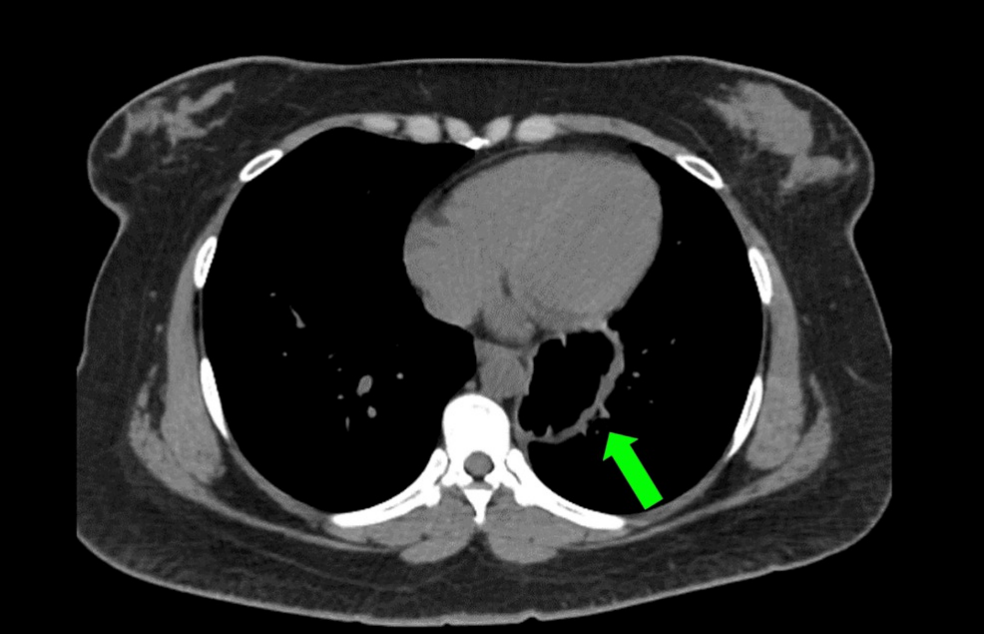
CT thorax axial view showing the herniation of body of stomach into the thoracic cavity (green arrow).
